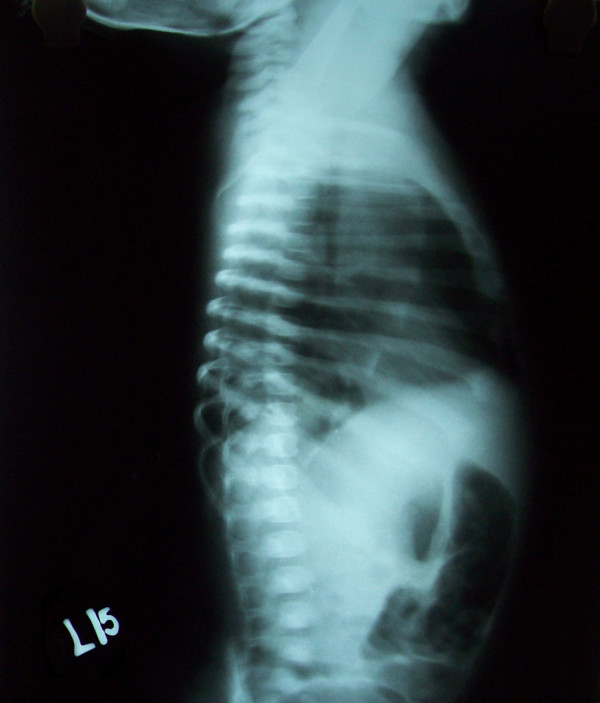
Left lateral view of Chest X-ray. On lateral view, the heart is displaced posteriorly with retrosternal luscency representing an anteriorly herniated lobe.
